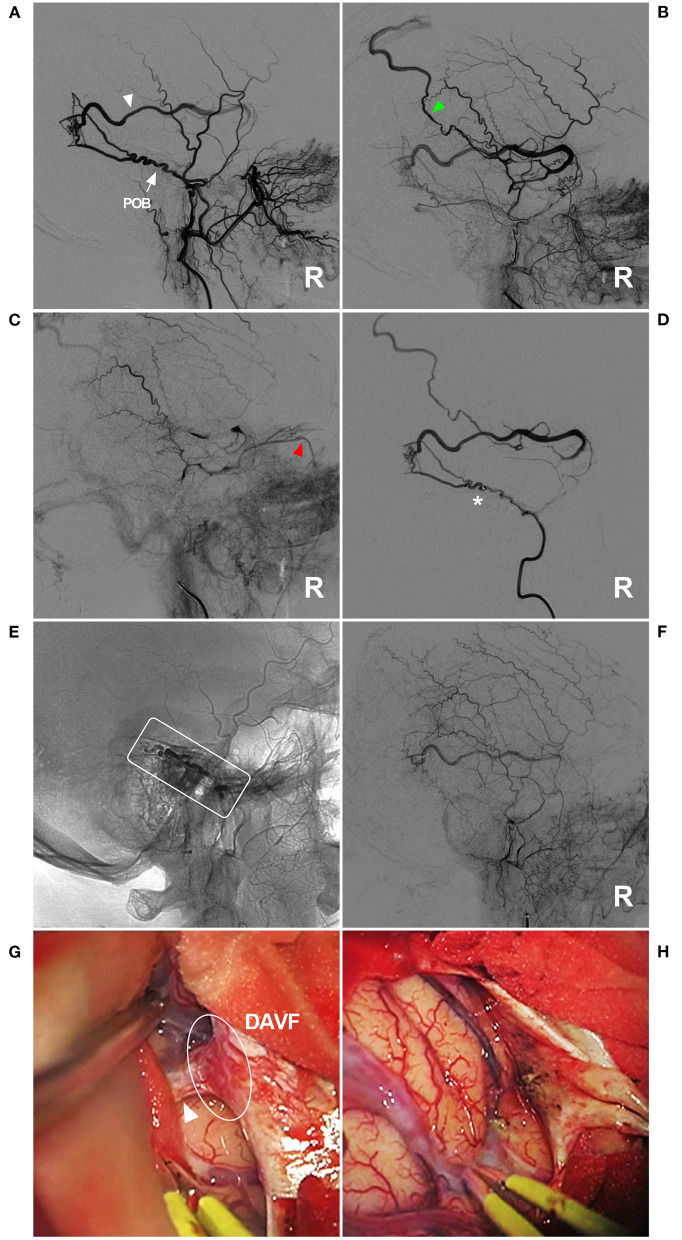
Unsuccessful type IIb EVT for a DAVF in the temporal region. (A–C) Lateral view ECA DSA showed a DAVF in the temporal region supplied by a normally developed and tortuous POB (arrow) (A). The draining vein behind the Sylvian fissure is clearly shown [white triangle in panel (A), early arterial phase] draining to the superior sagittal sinus [green triangle in panel (B), early arterial phase] and the ophthalmic vein [red triangle in panel (C), late arterial phase]. (D) Right MMA angiography showed that the microcatheter could not pass through the tortuous POB (asterisk). (E) X-ray film showing that Onyx (frame) could not reach the point of the fistula. (F) DSA showing the incomplete EVT. (G) Intraoperative imaging showing the DAVF (ellipse) and the draining vein (triangle). (H) The DAVF and draining vein were coagulated and cut. DAVF, dural arteriovenous fistula; DSA, digital subtraction angiography; ECA, external carotid artery; EVT, endovascular treatment; MMA, middle meningeal artery; POB, parieto-occipital branch; R, right.
